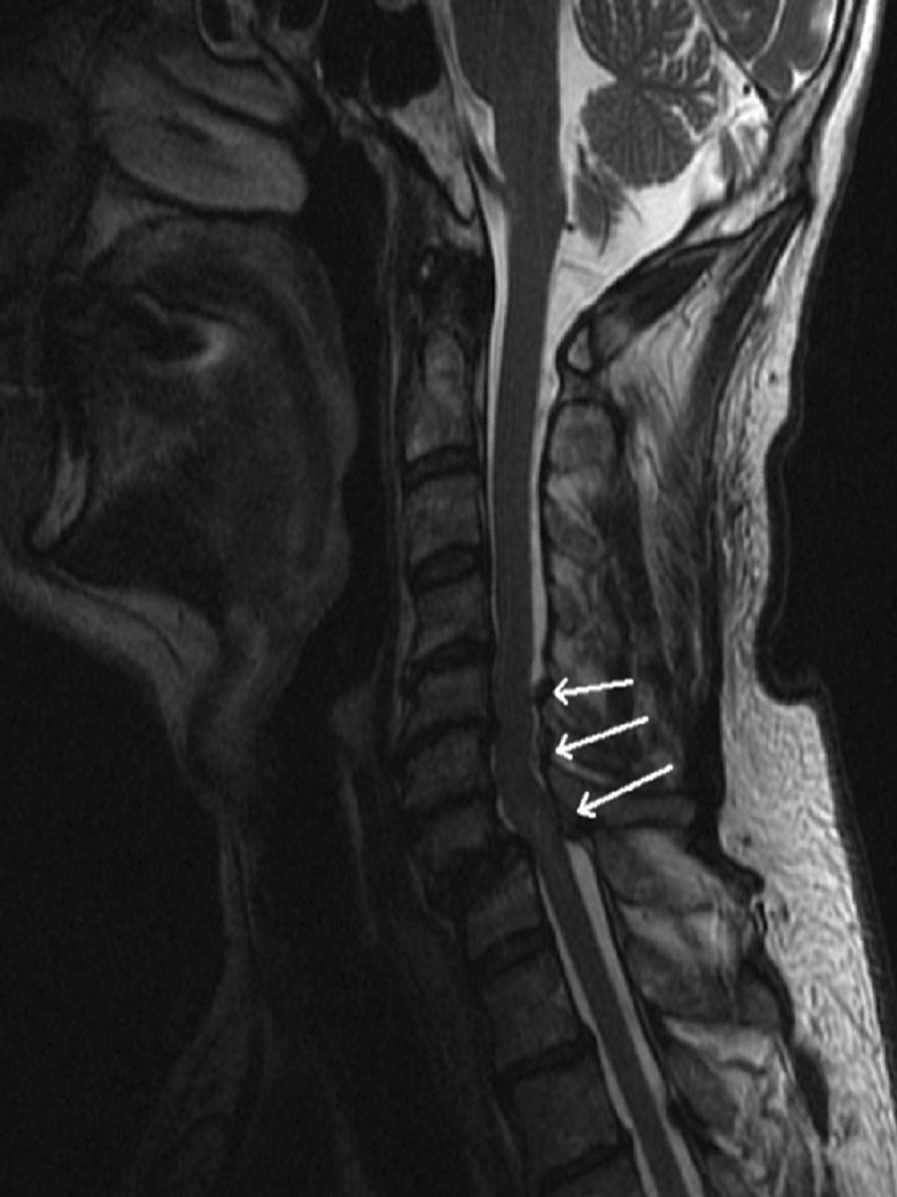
Magnetic resonance imaging of the cervical spine shows a compression of the spinal cord at C5-C6, C6-C7 und C7-T1 (preoperative).
